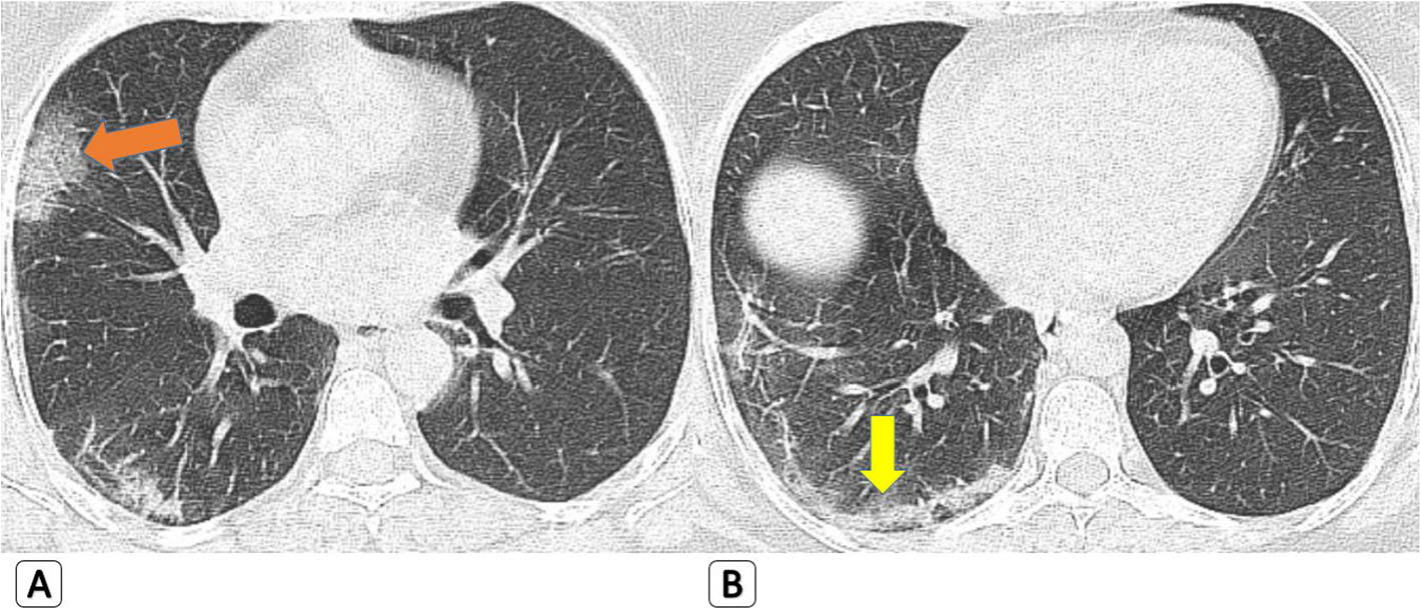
Mixed GGOs and “curvilinear organizing pattern of consolidation” seen with COVID-19. a, b 43-year-old female *COVID-19 patient* with axial HRCT chest lung window showing right sided upper lobar sub-pleural ground glass patchy showing “crazy paving pattern” (orange arrow) and lower lobar sub-pleural curvilinear pattern of organizing consolidation parallel to the pleural lining (yellow arrow)
